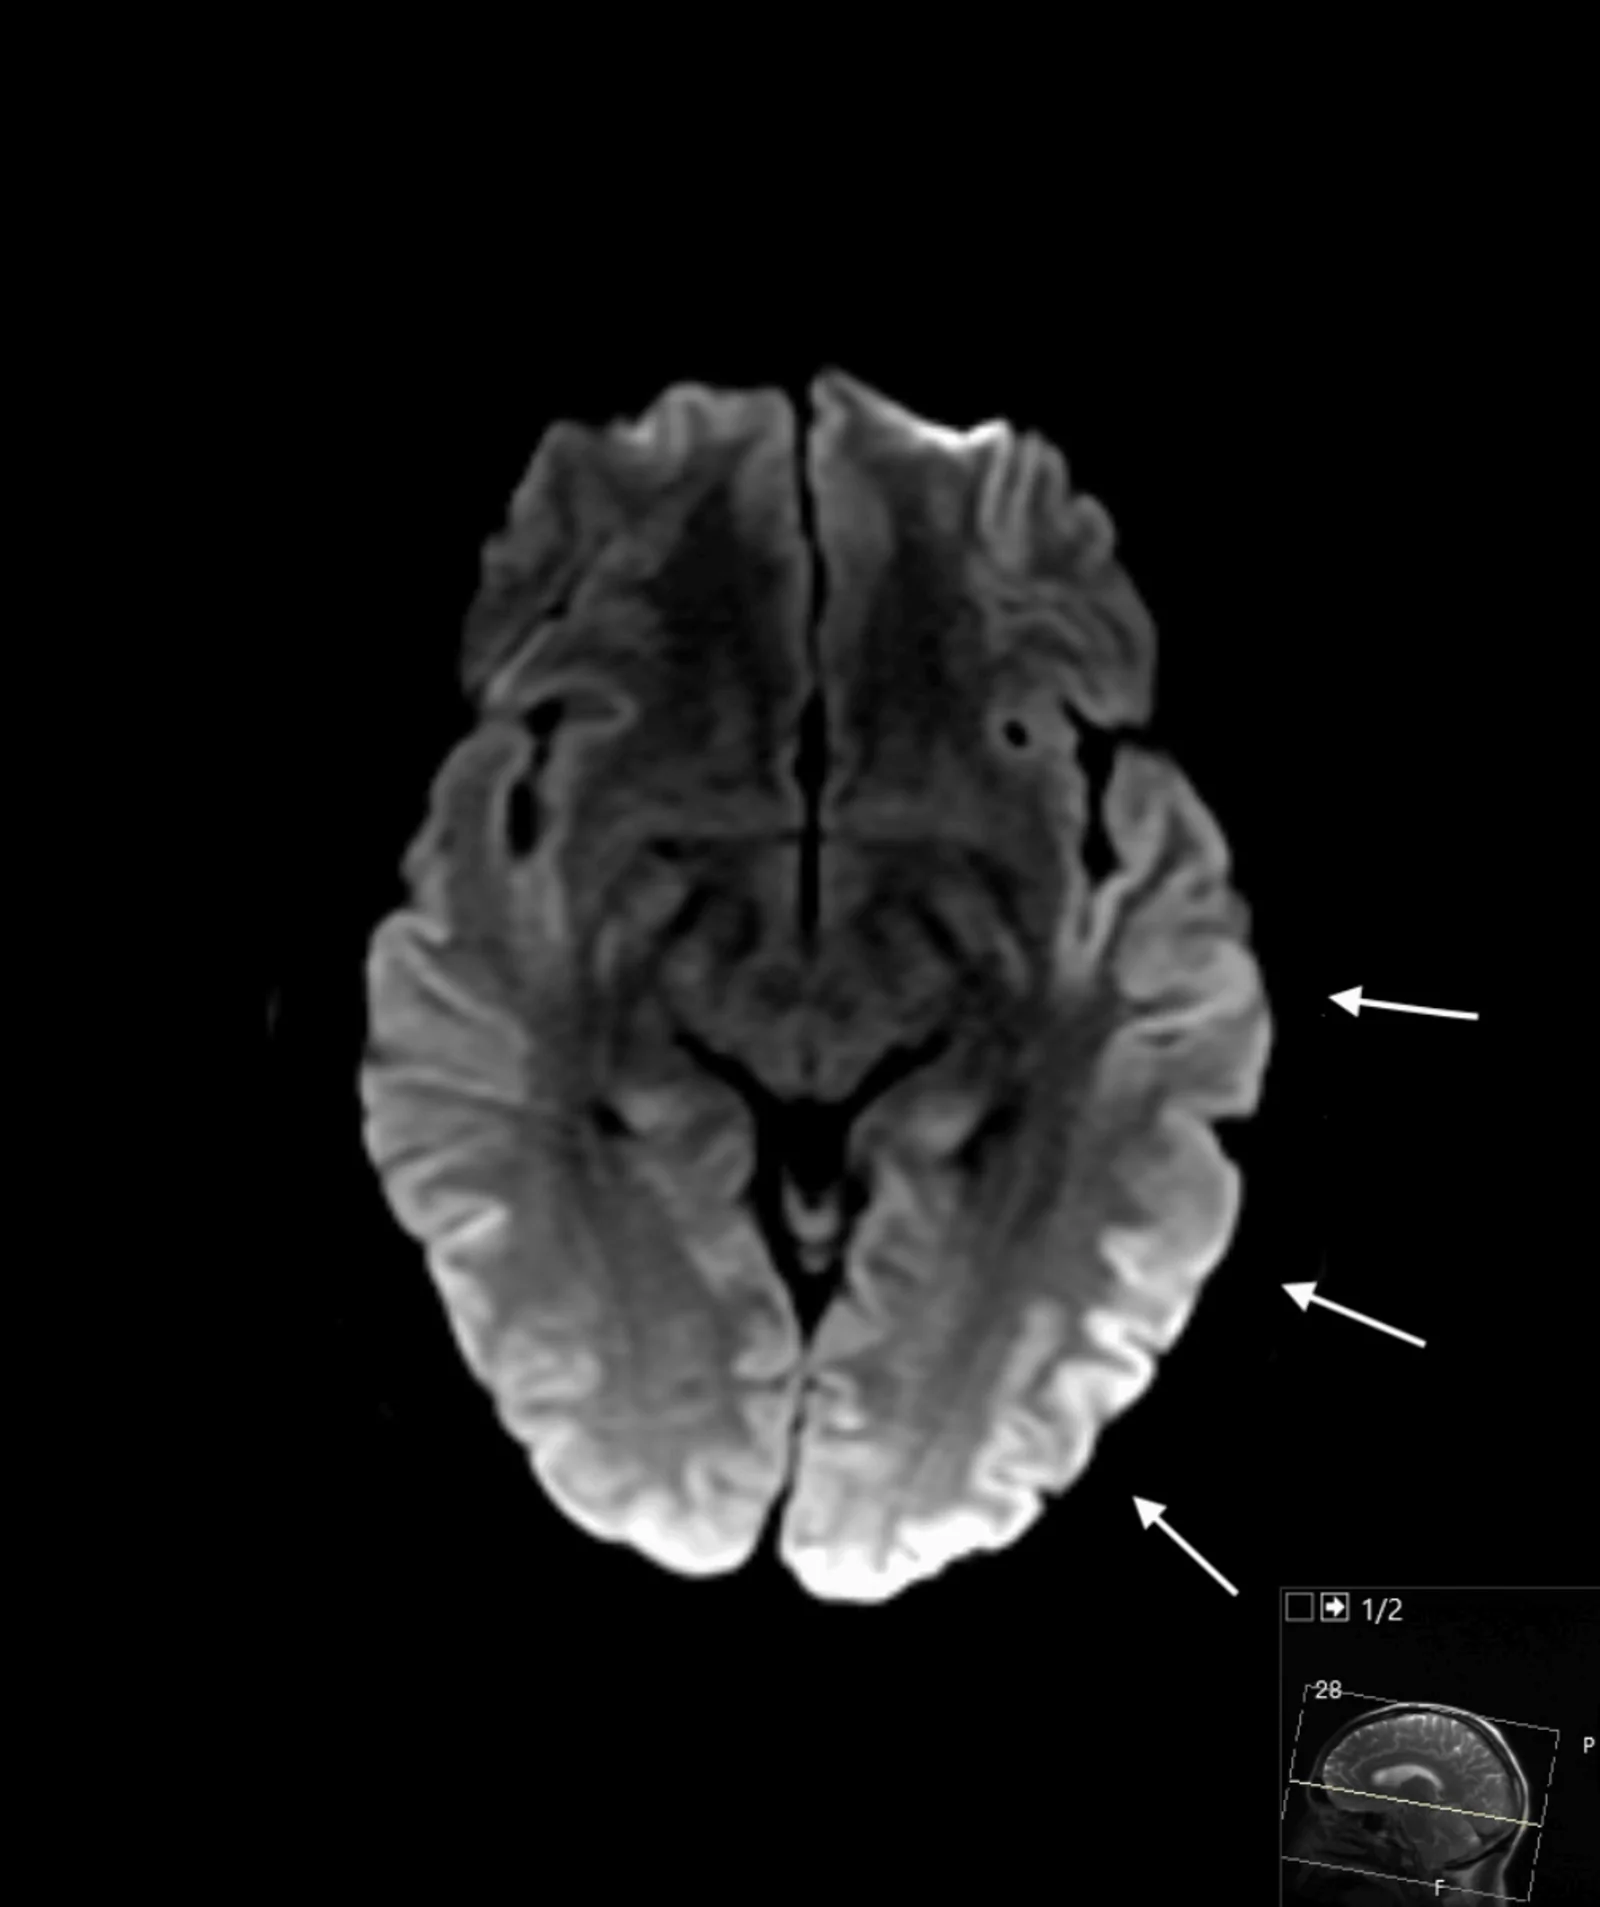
Axial diffusion-weighted imaging (DWI) of the brain demonstrating cortical ribboning with high signal intensity predominantly involving the left hemispheric cortex (arrows), consistent with Creutzfeldt-Jakob disease
